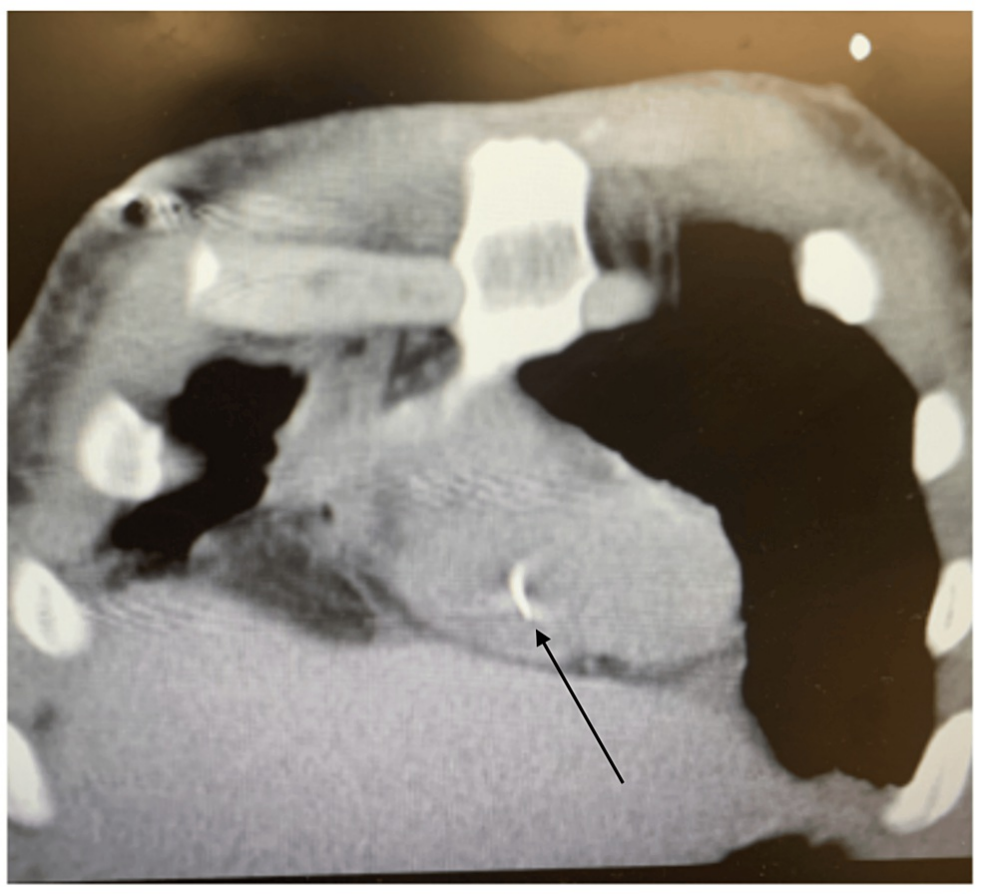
Chest CT coronal view showing a radio-opaque foreign object in the right ventricle (black arrow).
